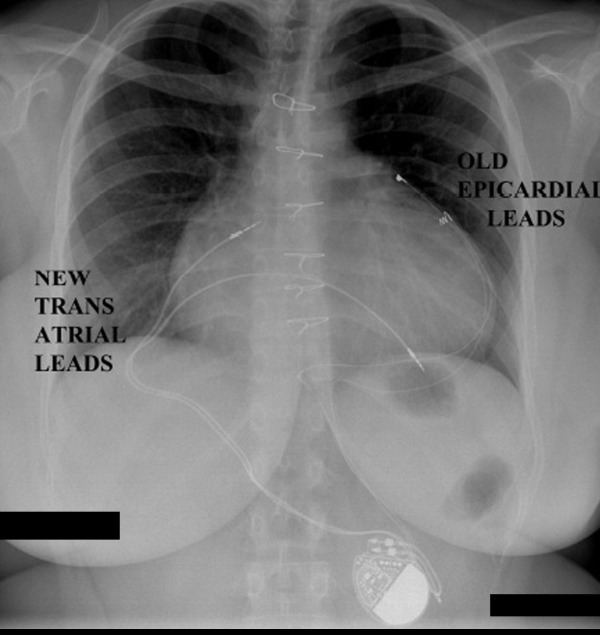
Figure 2 shows chest X-ray demonstration of both the old epicardial dual pacing leads and new transatrial endocardial screw-in leads; the pulsegenerator is located in the subxiphoid epigastric subcutaneous pocket.
